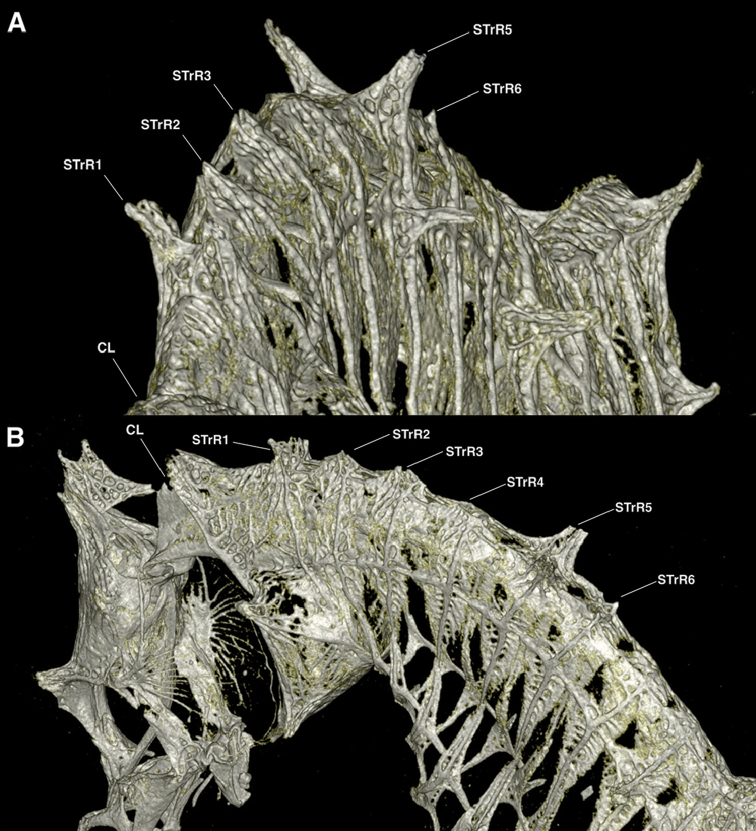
Computed tomography scanned anterior trunk area of *Hippocampusjapapigu*, UW 157506, male holotype, 16.33 mm SL, Hachijo-jima Island, Japan (photograph Graham Short). A Anterolateral view B Lateral view. Note the pair of spines projecting dorsolaterad on STrR1 and triangular bony mounds arched dorsad on STrR2, STrR3, and STrR4. Abbreviations: CL, cliethral ring; STrR1, first superior trunk ridge; STrR2, second superior trunk ridge; STrR3, third superior trunk ridge; STrR4, fourth superior trunk ridge; STrR5, fifth superior trunk ridge; STrR6, sixth superior trunk ridge.
